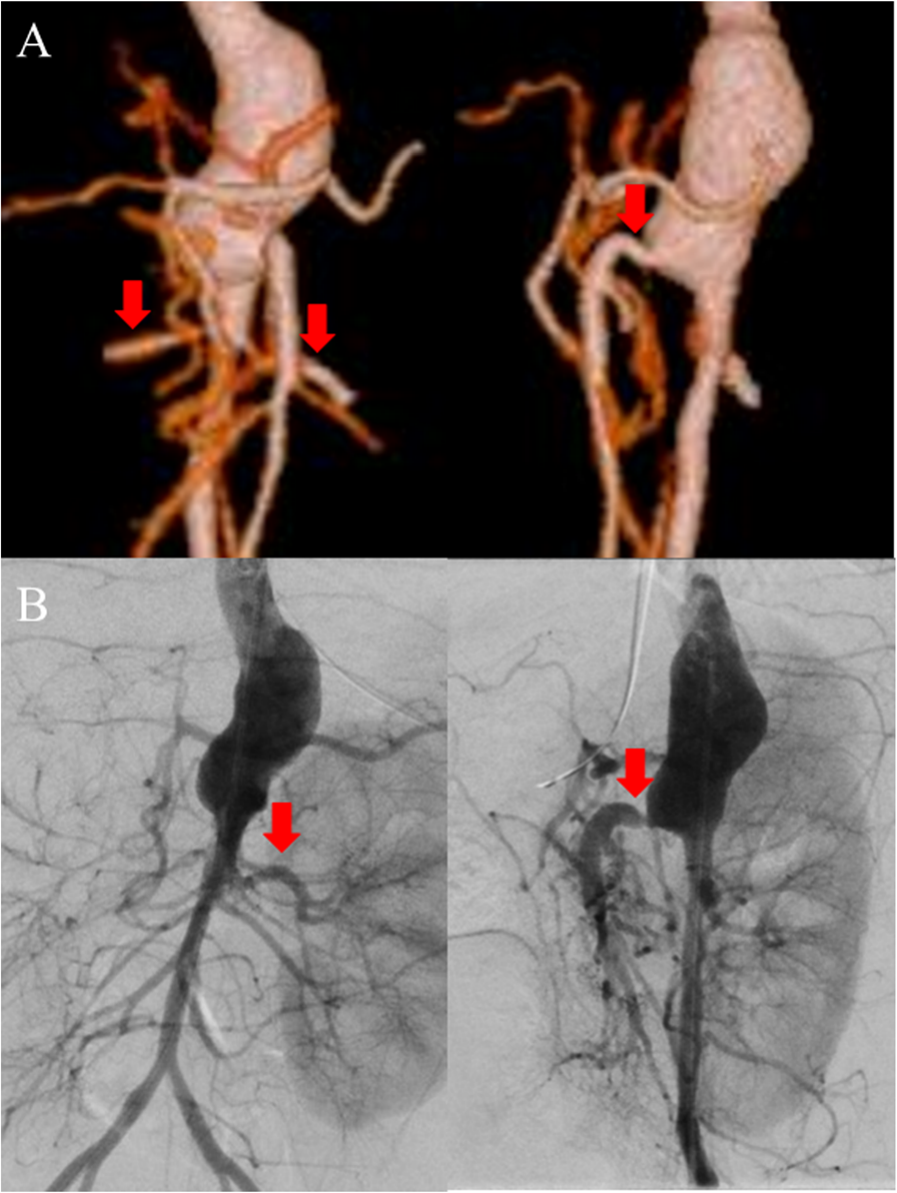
a Three-dimensional computed tomography on hospital day 3. Frontal (*left*) and left lateral (*right*) view; *arrows* show bilateral renal arteries (*left figure*) and superior mesenteric artery (*right figure*). b Angiographic image on hospital day 45. Frontal (*left*) and left lateral (*right*) view. The *arrow* shows the left renal artery (*left figure*) and superior mesenteric artery stenosis (*right figure*). Right renal artery is not contrasted
